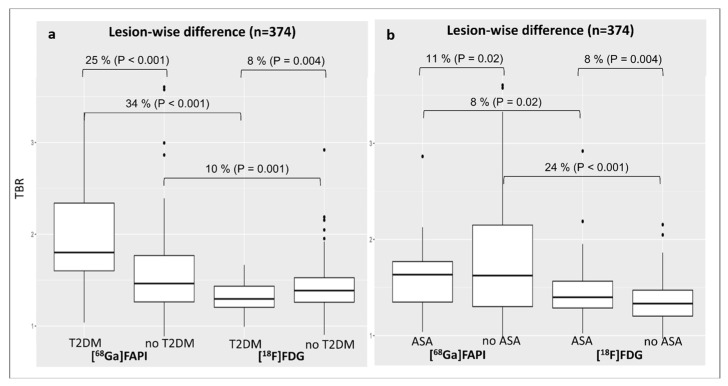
Changes in lesional TBR values for both tracers according to the diagnosis of T2DM (a) or the intake of ASA (b). ASA, acetylsalicylic acid; [^68^Ga]Ga-FAPI, [^68^Ga]-labeled fibroblast activation protein inhibitor; [^18^F]FDG, 2-deoxy-2-[^18^F]fluoro-D-glucose; TBR, target-to-background ratio; T2DM, type 2 diabetes mellitus.
